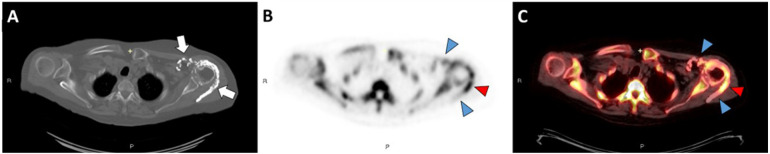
Axial CT image in bone windows (A) demonstrates periarticular calcinosis of the left shoulder involving the rotator cuff interposed between the deltoid and infraspinatus muscles (white arrows). Axial ^18^F-NaF PET/CT images (B,C) demonstrate intense activity at the mid/distal component of the calcification (red arrowheads) and mild activity along the proximal component and anterior calcifications (blue arrowheads). There is also increased ^18^F-NaF uptake at the left shoulder compared to the right, suggesting arthritic remodeling.
